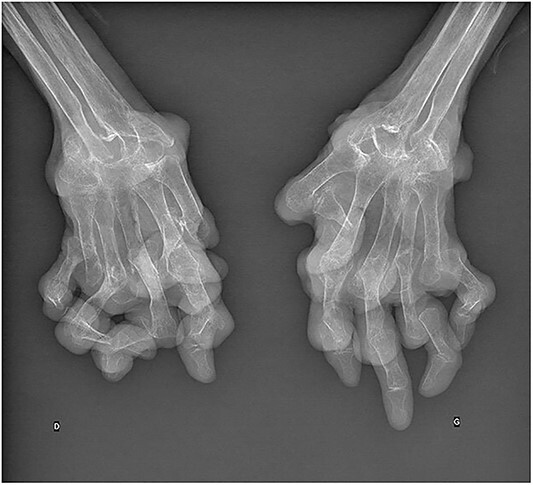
Radiographs of both hands showing subluxations in the metacarpophalangeal and proximal interphalangeal joints, alongside extensive carpal destruction.
